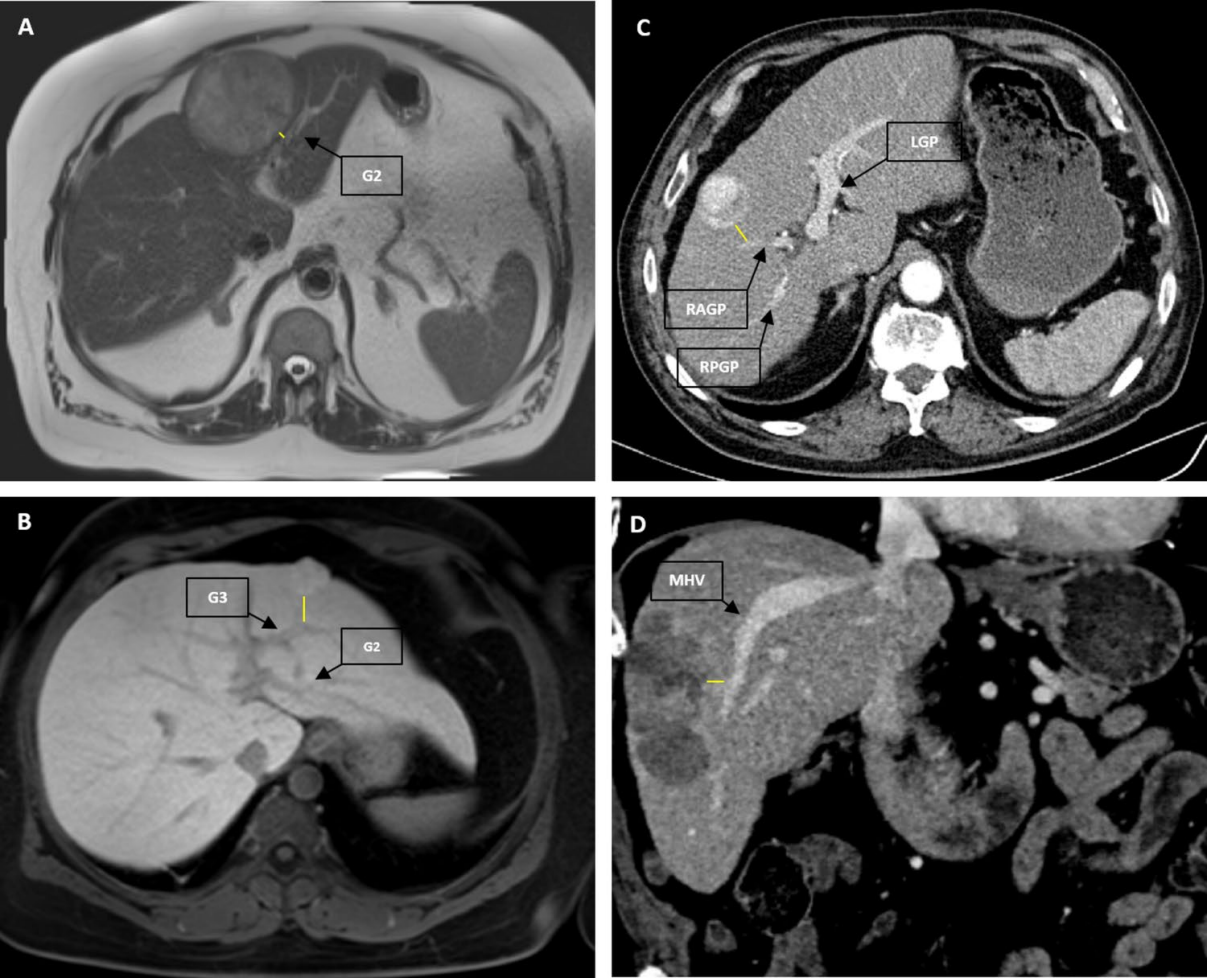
DTV-Measurement based on preoperative Imaging. Hepatic preoperative MRI (A-B) and CT (C-D). A: T2-weighted, B: Fat saturated T1-weighted. C: Arterial phase, D: Portal venous phase. DTV (yellow line) measured at the point of nearest distance between the tumor and any of the main hepatic vessels, such as the Glissonean pedicle (first- and second-order branches) or hepatic vein at caval confluence. *G2* Glissonean pedicle of segment 2; *G3* Glissonean pedicle of segment 3; *LGP* left Glissonean pedicle; *RAGP* right anterior Glissonean pedicle; *RPGP* right posterior Glissonean pedicle; *MHV* middle hepatic vein
